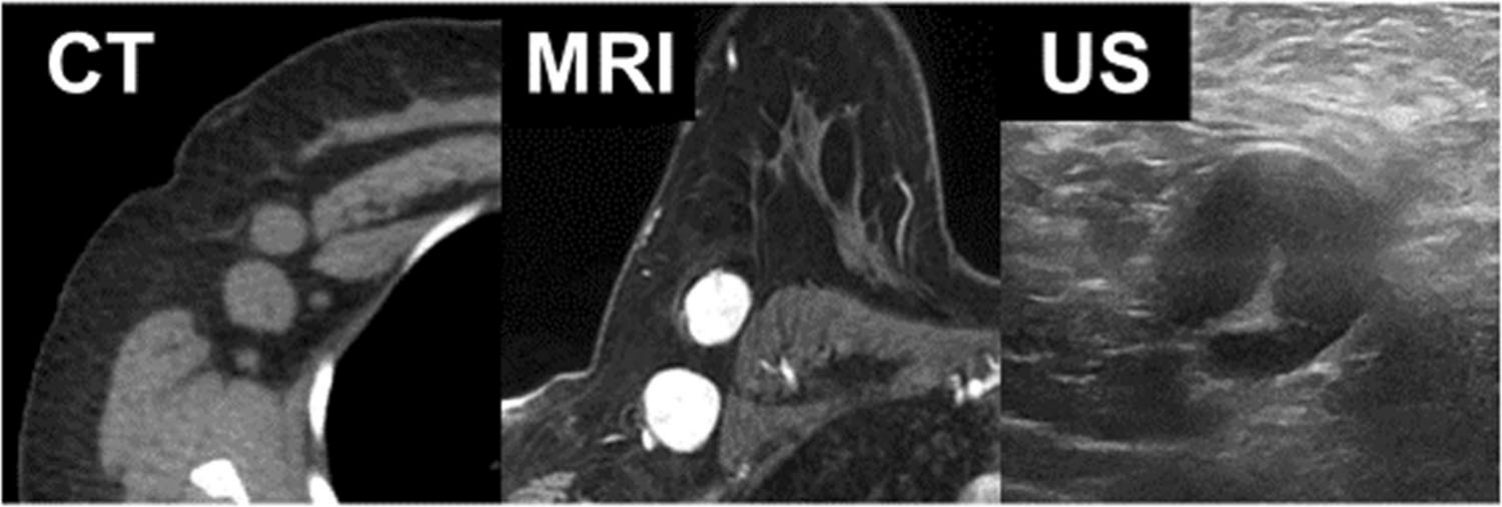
The same abnormal right axillary nodes by CT, MRI, and US in a breast cancer patient. All modalities demonstrate rounded rather than elongated shape, cortical thickening, and loss of the fatty hilum (a small residual fatty hilum is visualized by US)
